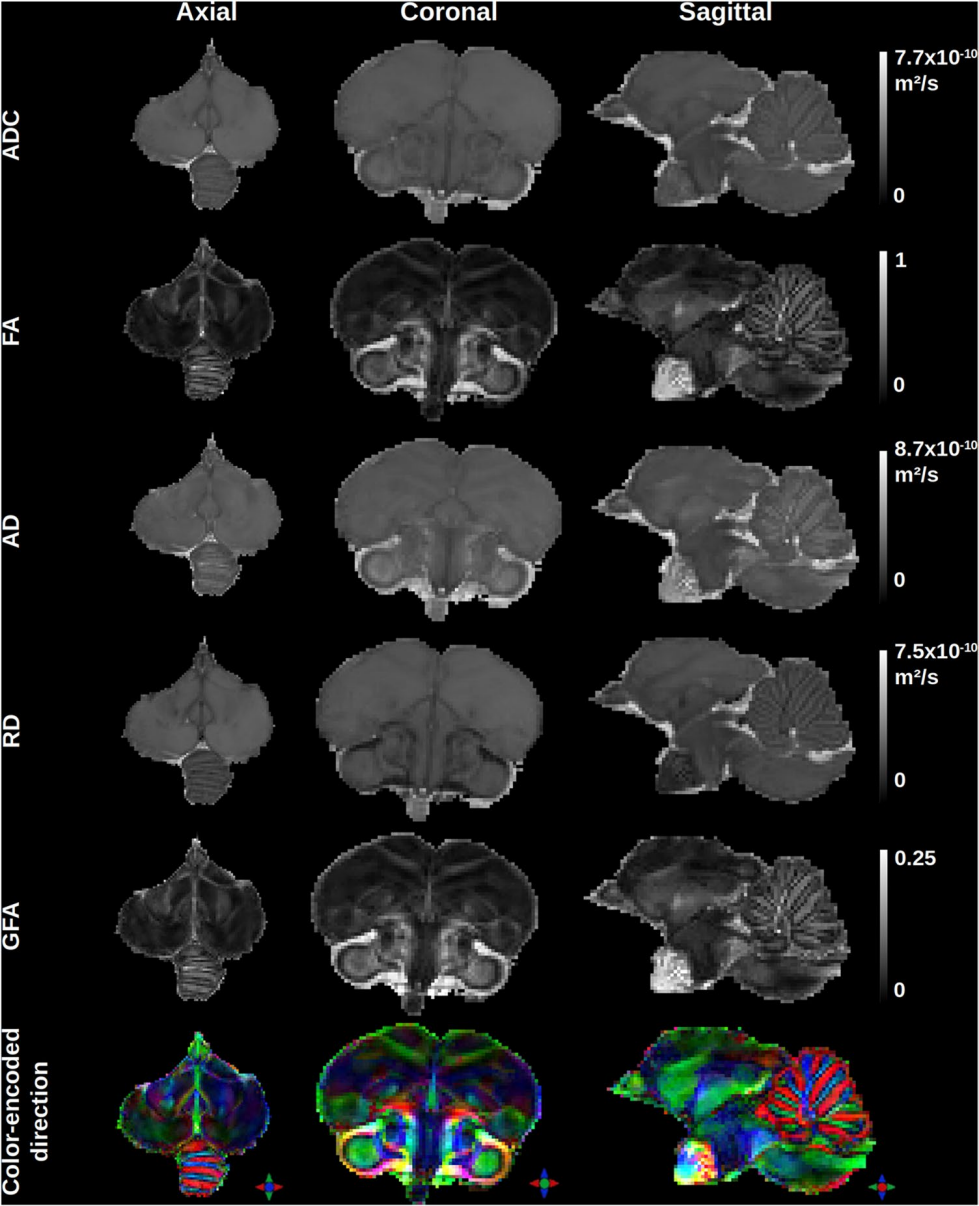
Representation of rotationally invariant DTI quantitative maps including (1st line) the apparent diffusion coefficient (ADC), (2nd line) the fractional anisotropy (FA), (3rd line) the axial diffusivity (AD), (4th line) the radial diffusivity (RD), and of analytical Q-ball quantitative maps including (5th lines) the generalized fractional anisotropy (GFA) and (6th line) the color-encoded direction (CED) map computed from a single-shell HARDI diffusion MRI acquisition performed on the brain of a Japanese quail at 11.7 T
